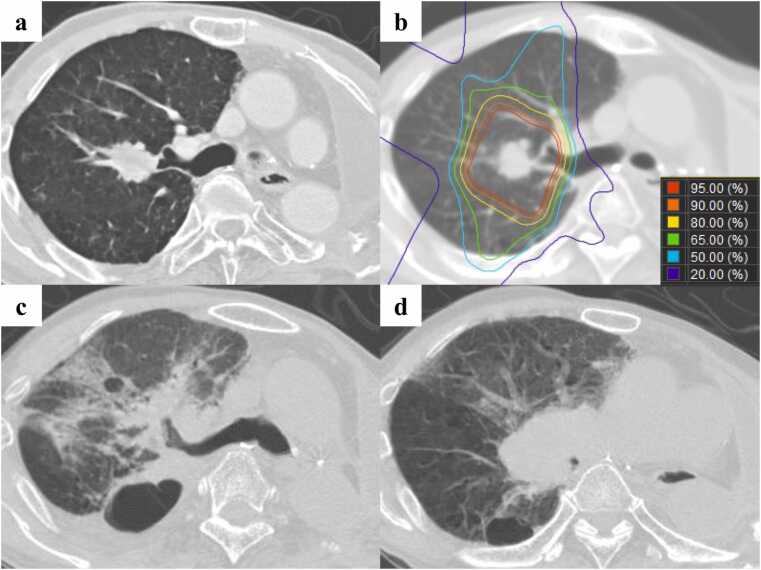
Computed tomography images of case 2. a) A primary tumor in the right S2 before proton beam therapy (PBT) b) The dose distribution of PBT. c) Radiation fibrosis without progression of the primary tumor at 12 months after PBT. d) Stenosis of the bronchus intermedius due to the right hilar lymph node recurrence at 12 months after PBT.
